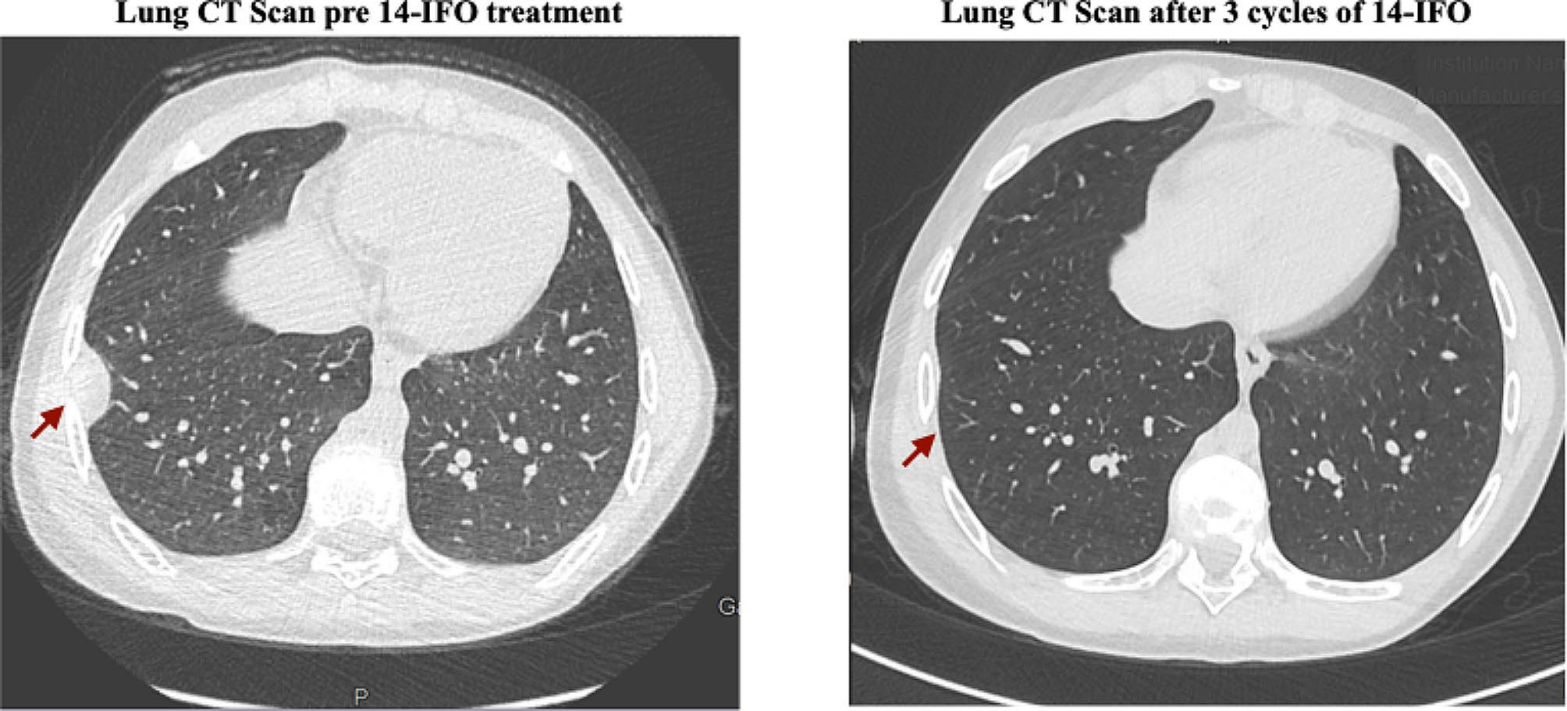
CT Scan images showing complete response according to RECIST Criteria v1.1 in a patient with relapses OS after 3 14-IFO cycles
